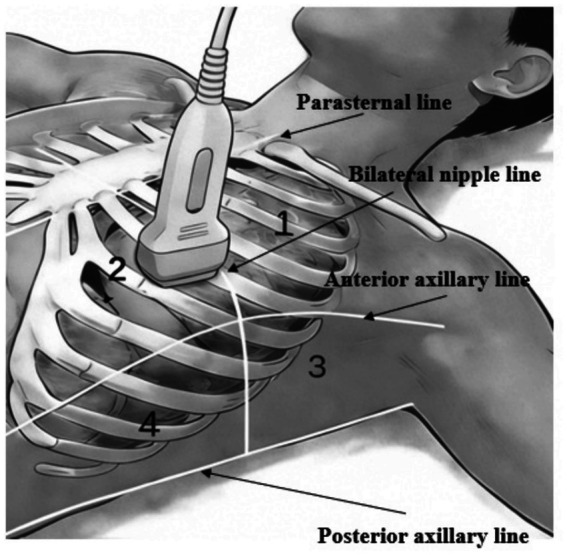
Regions in chest wall ultrasonographic examination. Based on the anatomical landmarks defined by the parasternal, anterior axillary, and posterior axillary lines, the hemithorax is divided into the anterior and lateral regions. Each region is further subdivided into superior and inferior subregions, bounded by the bilateral nipple line.
